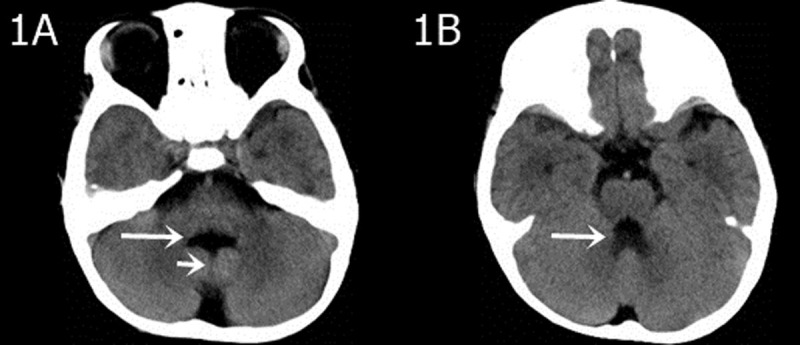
A three-year-old boy with a speech disorder and lifelong difficulty understanding simple instructions. A) A hypoplastic cerebellar vermis is evident on the axial CT image, with a midline fissure sign between the bilateral cerebellar hemispheres (short arrow), and a batwing sign on the fourth ventricle (long arrow). B) The upper cerebellar peduncles are thickened and elongated, forming a molar sign (arrow) with the midbrain.
